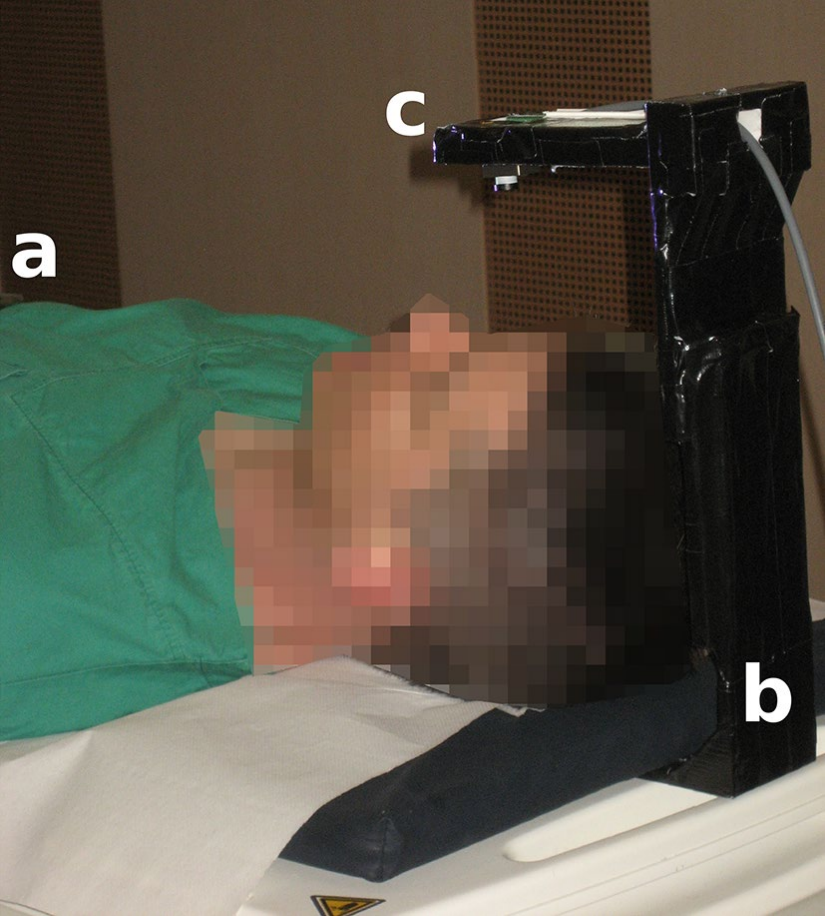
Hardware set-up of the proposed method. Set-up during experiments outside the bore showing a volunteer (a), the custom-built stand (b) and the MRI-compatible camera (c)
